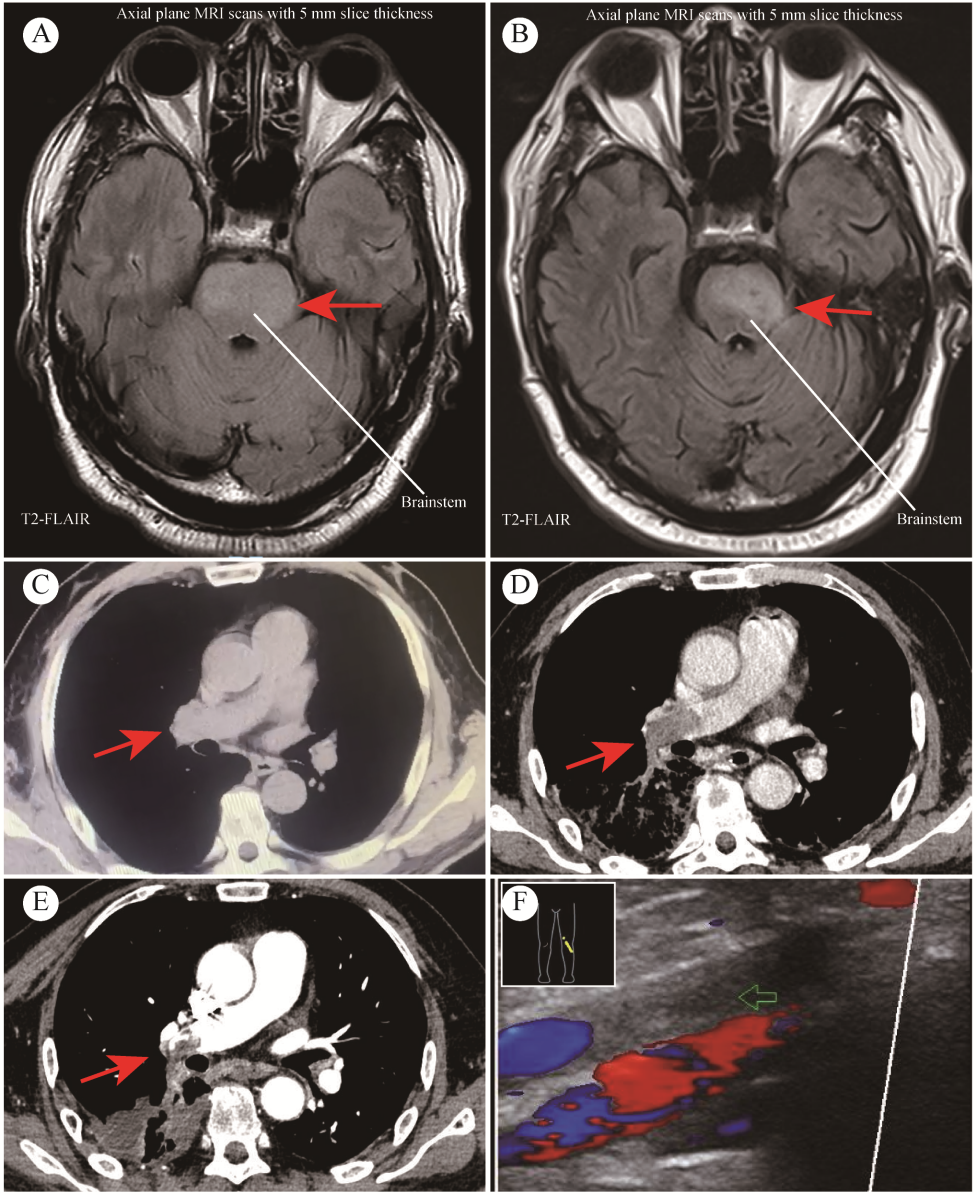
MRI and CT Imaging from case study. (A) Preoperative MRI findings; (B) MRI findings after 3 months of anlotinib treatment; (C) CT indicated no PE before initiating anlotinib treatment; (D) CT indicated extensive PE after 3 months of anlotinib treatment; (E) CT scan after 2 months of anticoagulant therapy; (F) Thromboses detected in lower leg veins.
